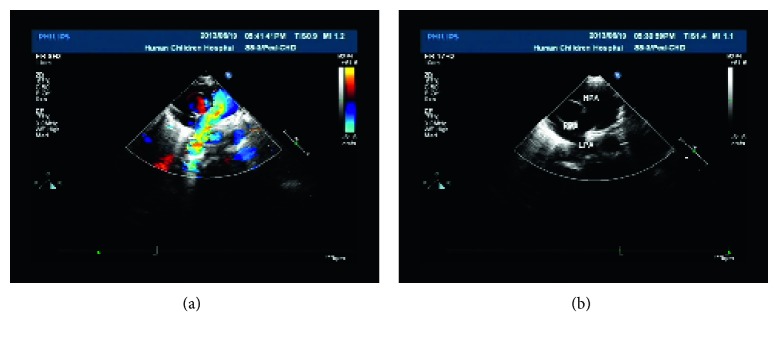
Echocardiograms showing PAS.
